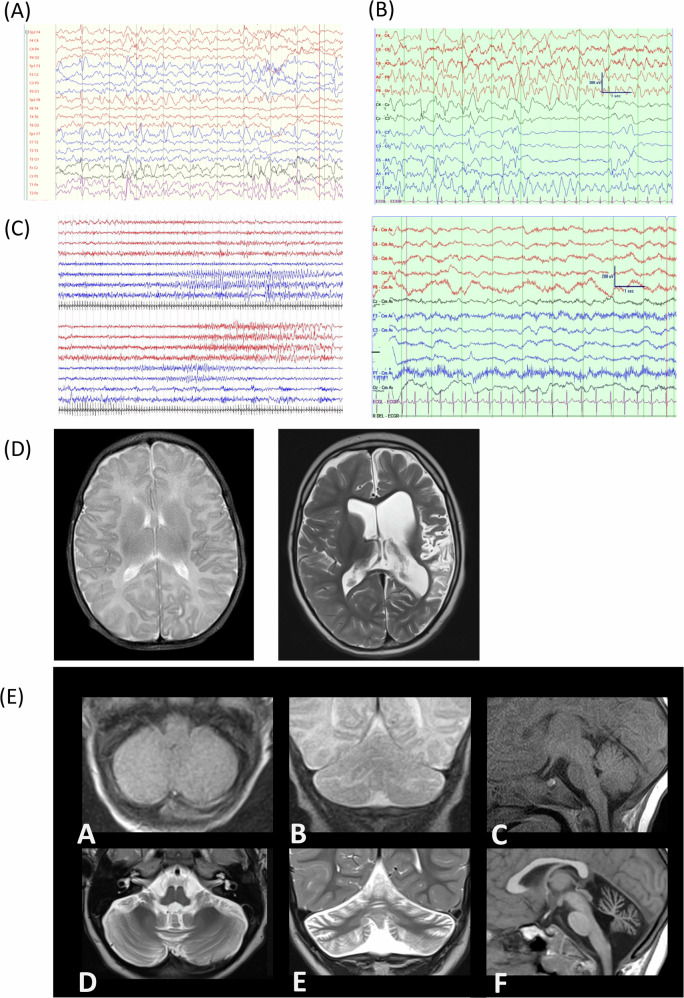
Electroencephalographic and neuroradiological findings. A Patient 12 – Interictal EEG demonstrating bilateral frontal spike-wave epileptiform activity, more prominent on the left. B Patient 3 – Ictal EEG demonstrating emergent amplitude asymmetry (R > L) evolving to asymmetrical left sided decrement associated with asynchronous clonic jerking. C Patient 2 – The left panel shows ictal EEG aged 4 months prior to lidocaine infusion. The EEG shows very frequent seizures in the left hemisphere (blue) spreading to the right hemisphere (red). The right panel shows EEG on intravenous lidocaine, capturing stiffening episodes with no EEG correlate. Epileptiform activity has disappeared. The background is slow as anticipated with sedation. D Patient 2 - Left hemisphere infarct. Axial MRI brain images show normal appearances aged 4 weeks (left) and evolution of a mature left hemisphere infarct aged 3 years (right). This is suspected the sequelae of super-refractory status epilepticus in the first year of life. E Patient 4 - Coronal and axial T2 weighted-images (A and B) and sagittal T1 weighted-images (C) when the patient was 1-month-old showing normal appearances of the posterior fossa structures. Follow-up magnetic resonance scan: coronal and axial T2 weighted-images (A and B) and sagittal T1 weighted-images (C) when the patient was 4 year-old showing development of global cerebellar atrophy without involvement of the pons and with normal cerebrum (“pure cerebellar atrophy”).
